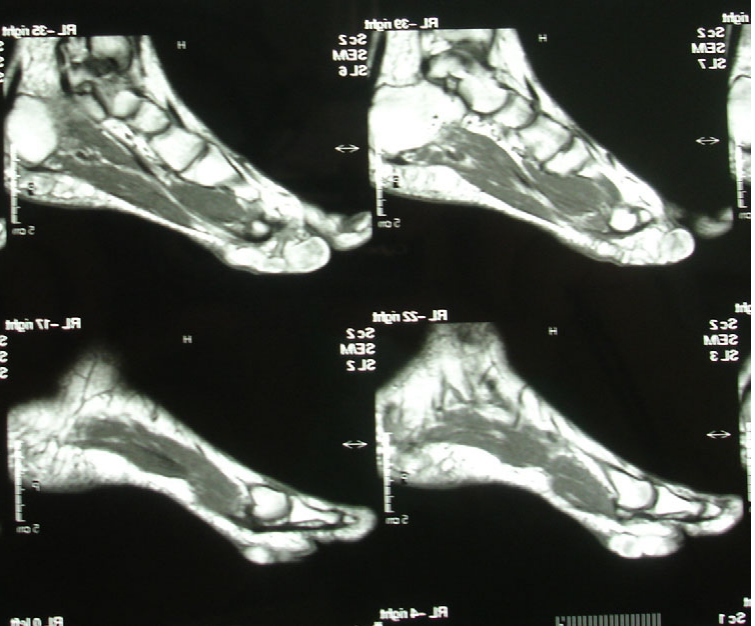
Magnetic resonance imaging (MRI) revealed a well-defined mass located at the plantar forefoot with no apparent bone infiltration.
